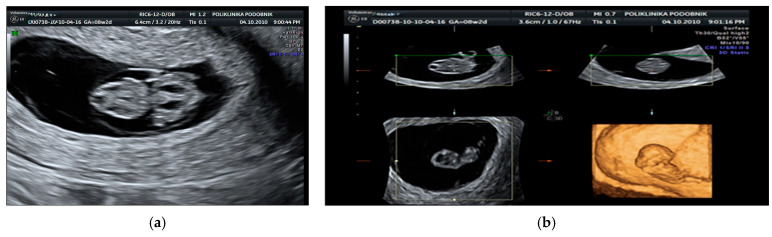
Longitudinal view of a non-septated cystic hygroma with associated hydrops fetalis and bilateral hydrothorax at 8.2 weeks of gestation. (a) Corresponding 3D transvaginal ultrasound image. (b) Fetal karyotyping revealed Turner syndrome. The pregnancy was electively terminated at the parents’ request.
